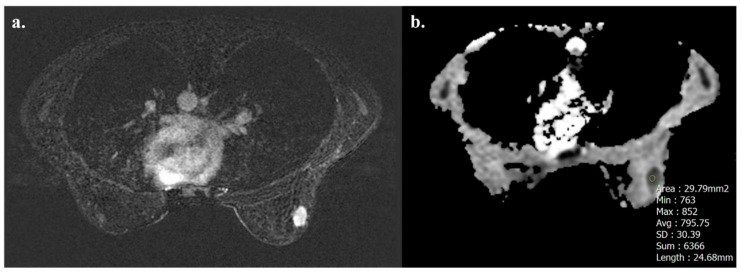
A 32-year-old woman with NST BC subtype Luminal B with high TIL levels (35%) in the right breast. Axial T1-weighted contrast-enhanced MR image shows homogeneously enhanced mass (a), and hypointensity on ADC map (0.795 × 10^−3^ mm^2^ s^−1^), as showed by the circle (b).
